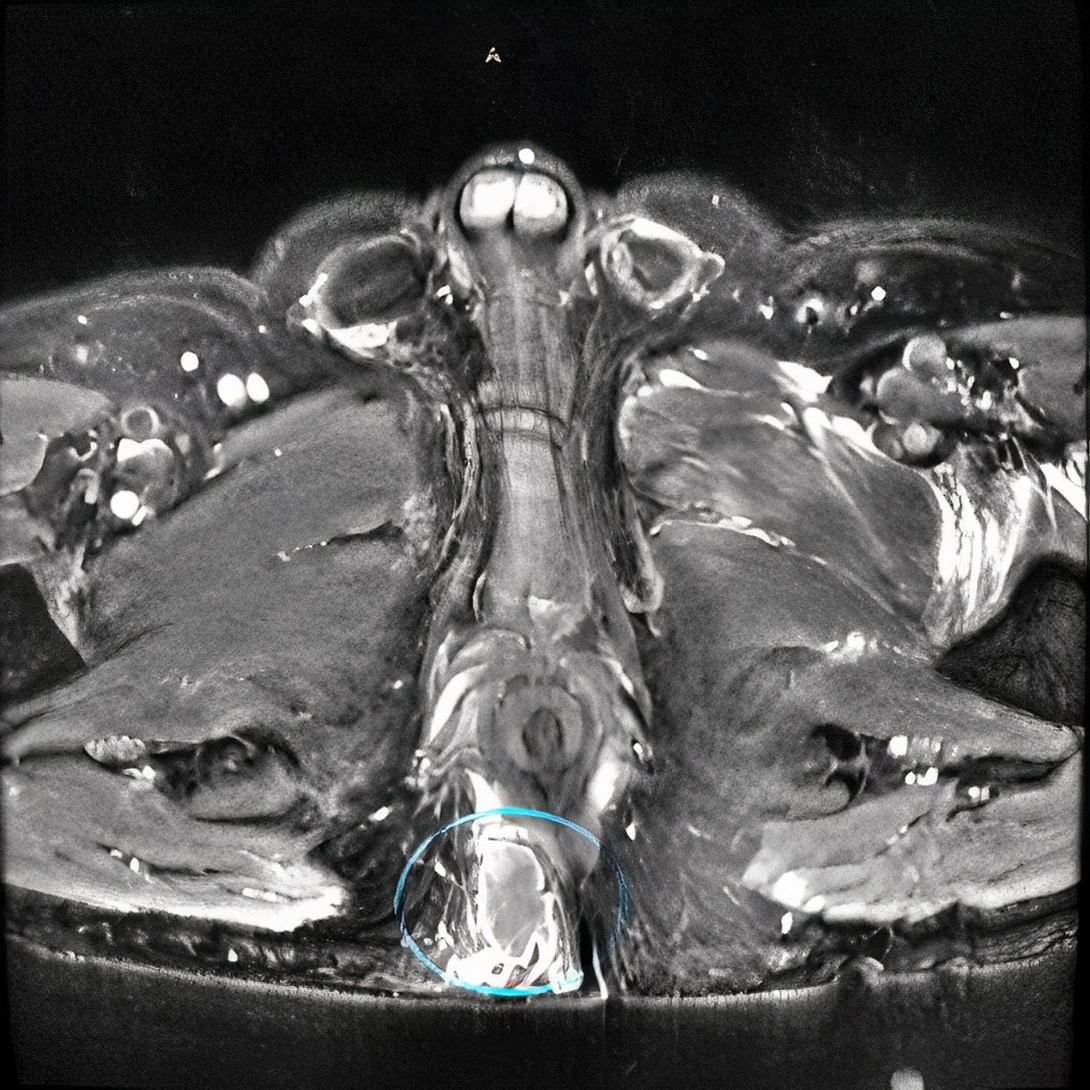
MRI abdomen/pelvis showing perirectal abscess (blue circle).
